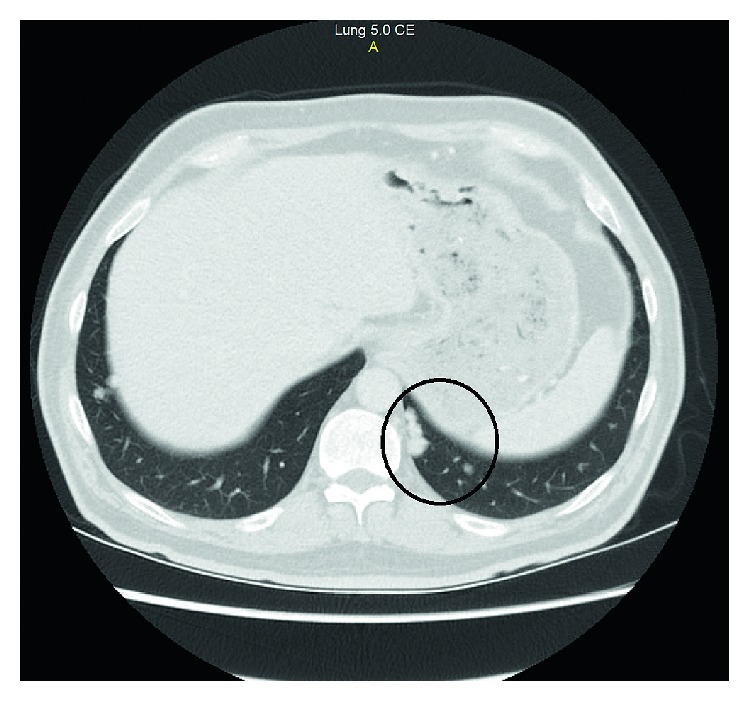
PET scan correlated CT section of lung nodule on day 45 of the vemurafenib treatment.
